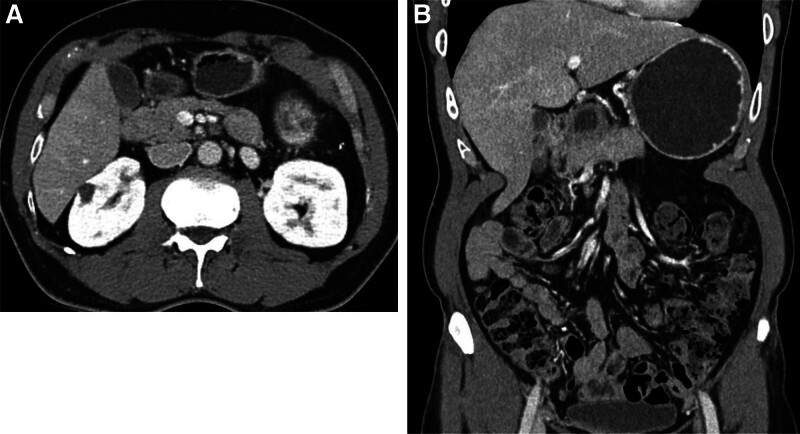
Postoperative follow-up CT. (A) Axial view of abdominal CT performed on May 10, 2024 (five months after surgery) showing resolution of previously engorged perigastric and splenic vein collaterals. (B) Coronal view confirming absence of abnormal varices or masses in the pelvic cavity 5 mo post-splenectomy. CT = computed tomography.
